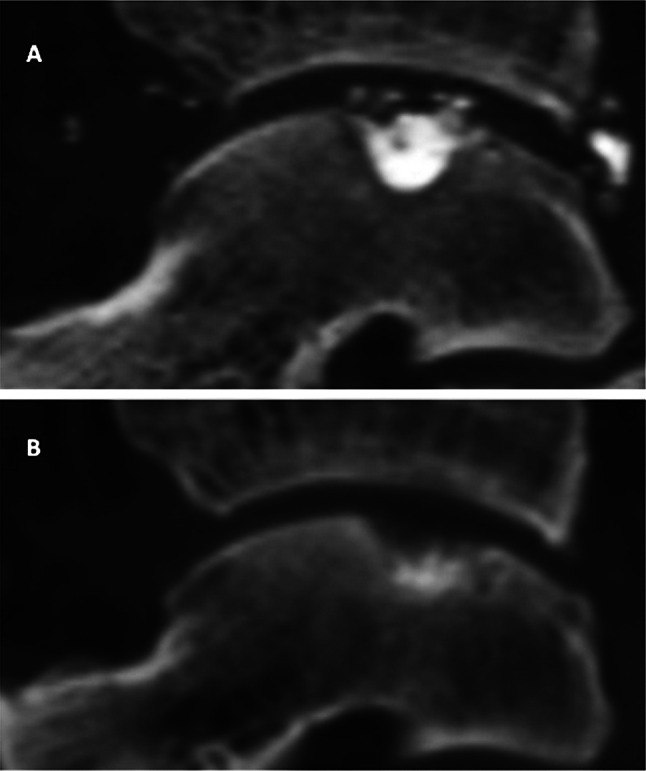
Post-operative computed tomography (CT) scans. a
